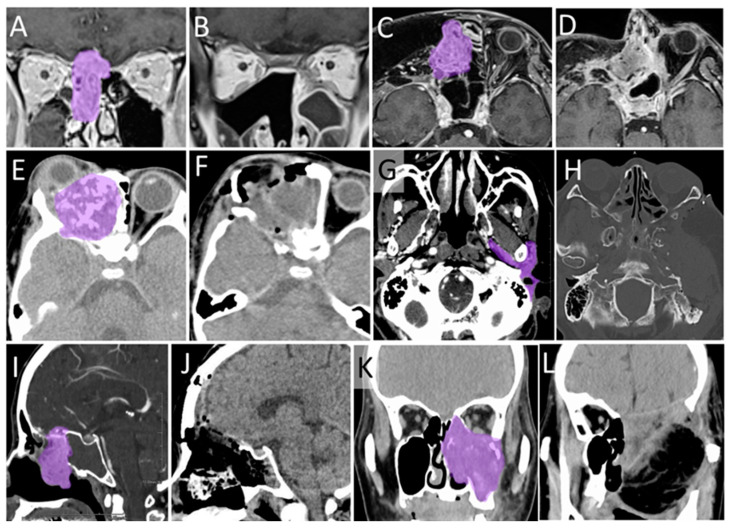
Pre- and postoperative images of Cases 3–8. (A,B) Gadolinium-enhanced T1 weighted coronal MRI images of Case 3, (C,D) Gadolinium-enhanced T1 weighted MRI images of Case 4, (E,F) CT images of Case 5, (G,H) CT images of Case 6, (I,J) Sagittal CT images of Case 7, (K,L) Coronal CT images of Case 8. (A,C,E,G,I,K) Preoperative images, (B,D,F,H,J,L) postoperative images. Purple; tumor. MRI, magnetic resonance imaging; CT, computed tomography.
